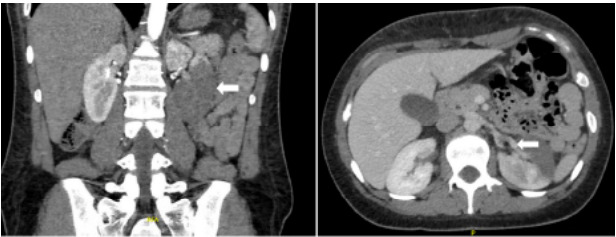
Left renal artery thrombosis with left kidney infarcts indicated by white arrow on computed tomography angiography—coronal view on the left and axial view on the right—done in the setting of epigastric pain.
